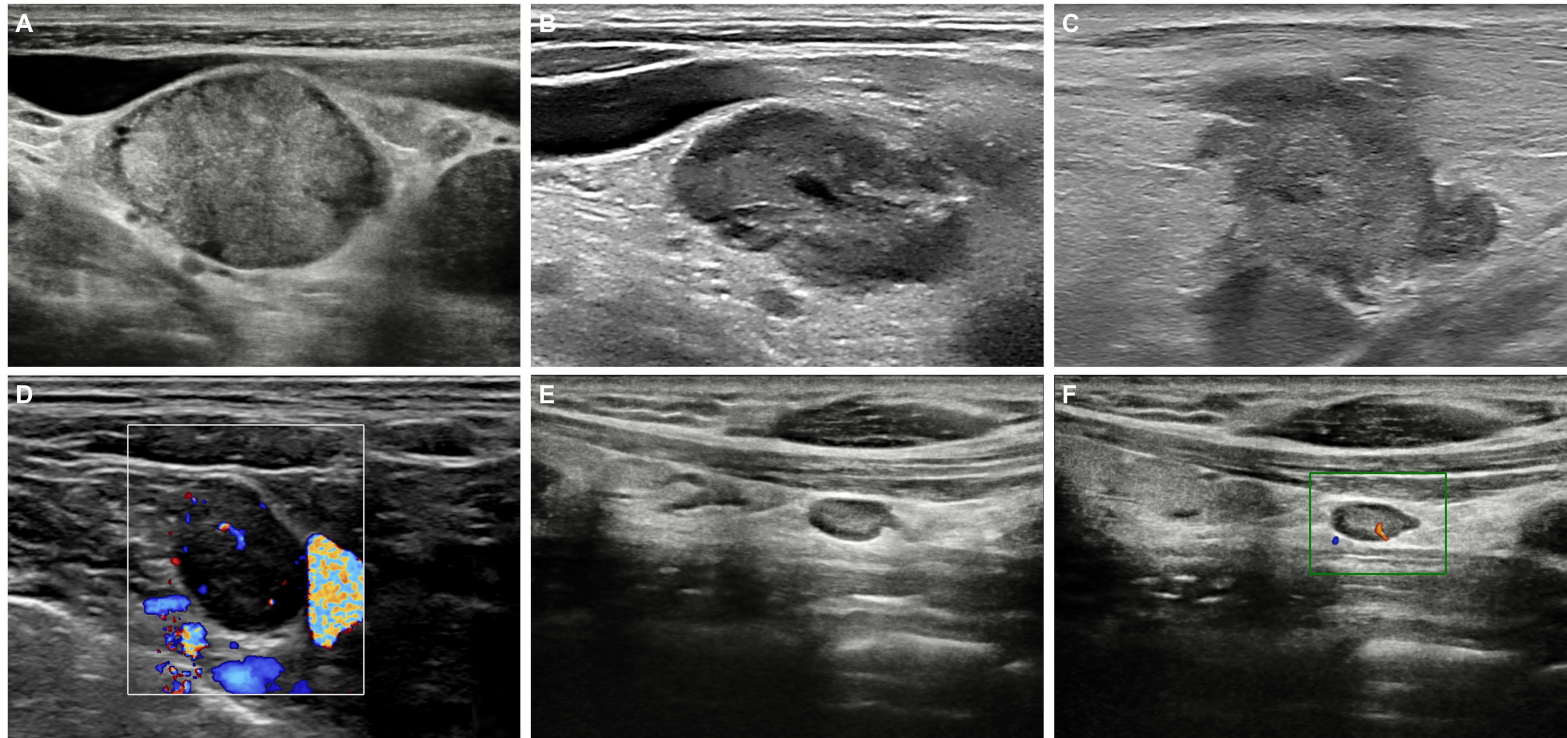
Ultrasound features of cervical metastatic and normal lymph nodes in esophageal cancer. (A) Metastatic lymph nodes have a short-axis diameter > 5 mm, appear hypoechoic, and are round in shape (long-to-short axis ratio ≤1), lacking a visible hilum structure. (B) Metastatic lymph nodes may contain areas of liquefaction or necrosis. (C) The borders of metastatic lymph nodes are often irregular. (D) Color Doppler Flow Imaging CDFI of metastatic lymph nodes shows abundant blood flow signals. Normal or reactive lymph nodes on B-mode (E) and CDFI (F).
